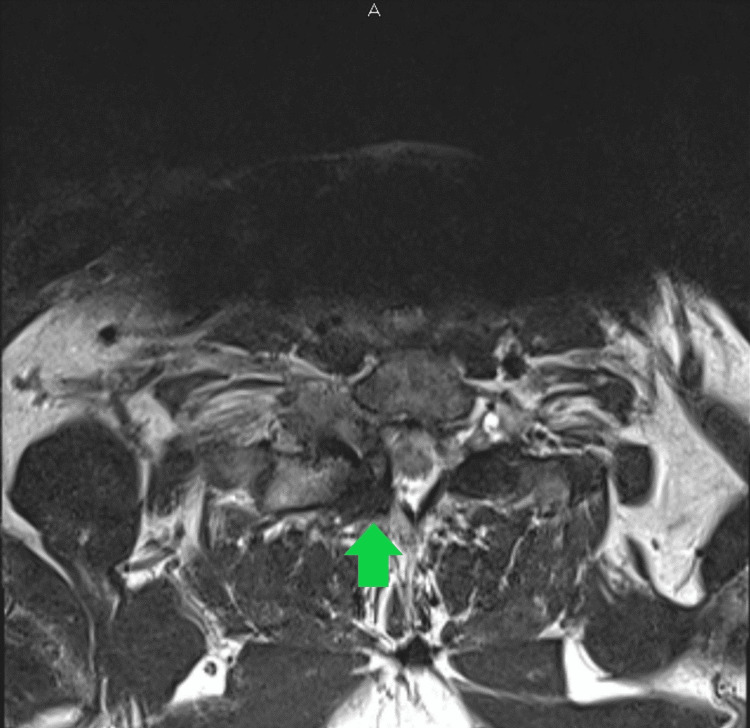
Axial MRI demonstrating the cyst and its relationship with the spinal cord.
